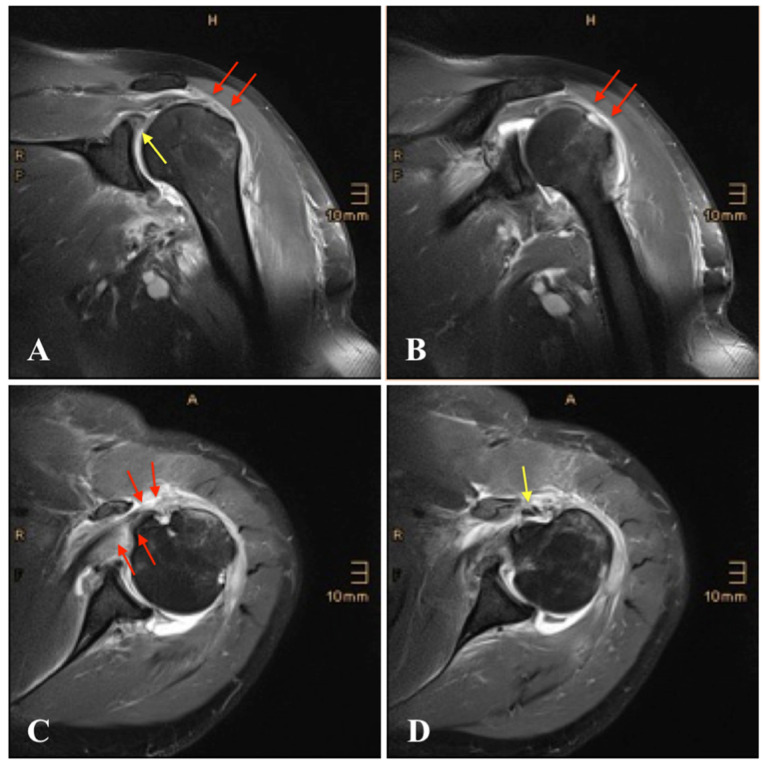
Magnetic resonance imaging (MRI) of the left shoulder following inferior dislocation: (A), coronal view with tear of the supraspinatus tendon (red arrows) and superior labrum anterior and posterior (SLAP) lesion (yellow arrow); (B), coronal view with tear of the infraspinatus tendon (red arrows); (C), axial view with tear of the subscapularis tendon and fatty degeneration of the muscle (red arrows); (D), axial view with rupture of the long head of the biceps tendon (LHBT) (yellow arrow).
